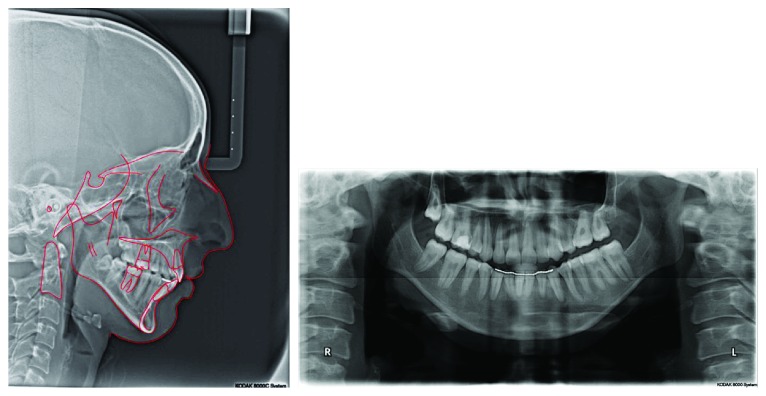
Posttreatment cephalometric and panoramic radiograph.
